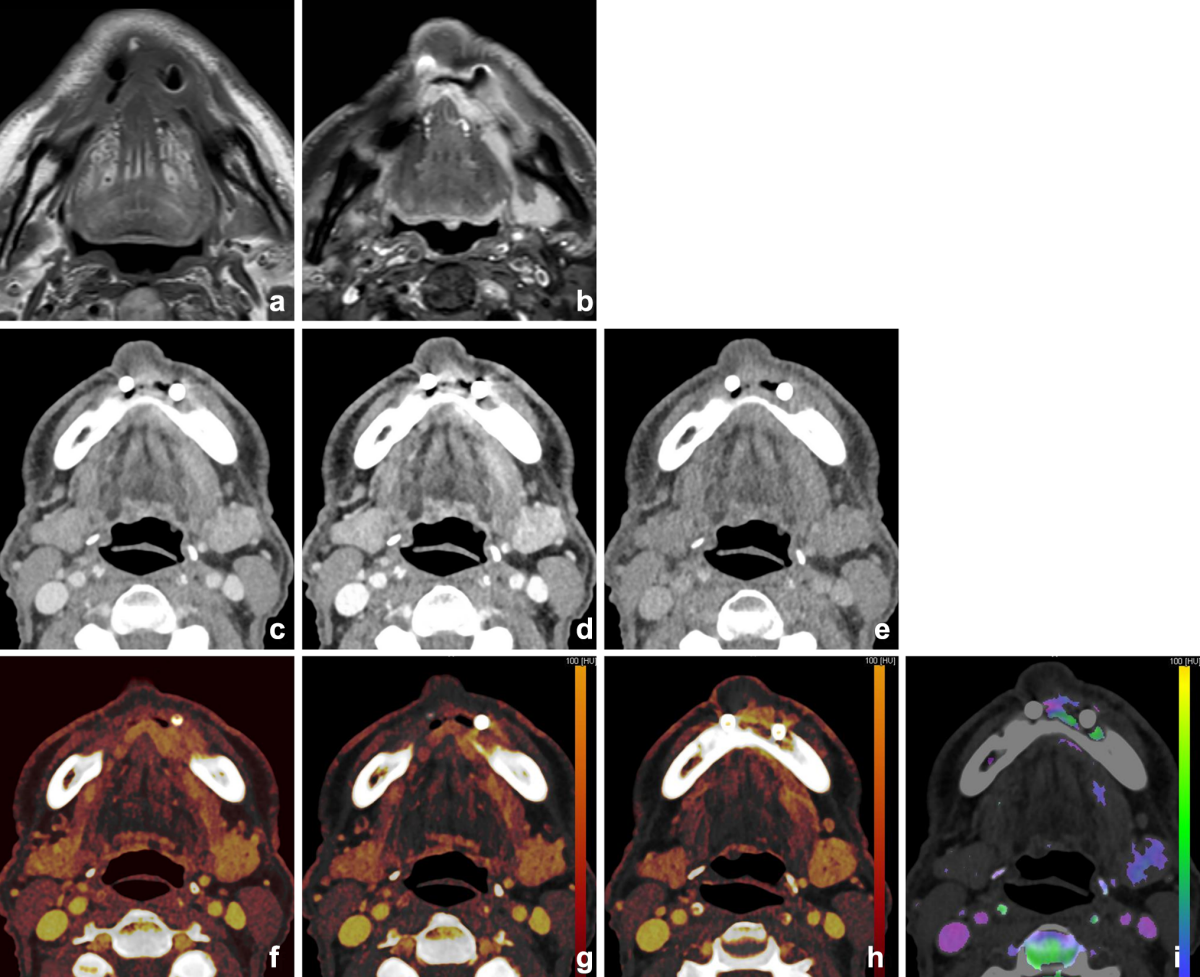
A male with previous laryngeal cancer and oral implants presented with suspicion of oral cavity SCC at the site of the implants. MR showed considerable distortion and interpretation difficulties at the tumor site (T1-weighted (T1-w) (a). Fat-supressed T1-w after gadolinium (b) demonstrated abnormal signal and enhancement; however, delineation was challenging due to artifacts. 5 mm DECT with soft kernel at 50% linear blending (c), 90% (d), and 10% (e) blending demonstrated the implants and osseous destruction of the mandible, better visualized at higher energies. Tumor enhancement was, however, difficult at mixed imaging, but was easily visualized at iodine fusion imaging (f–h). Bone marrow edema is demonstrated at BME imaging (i)
